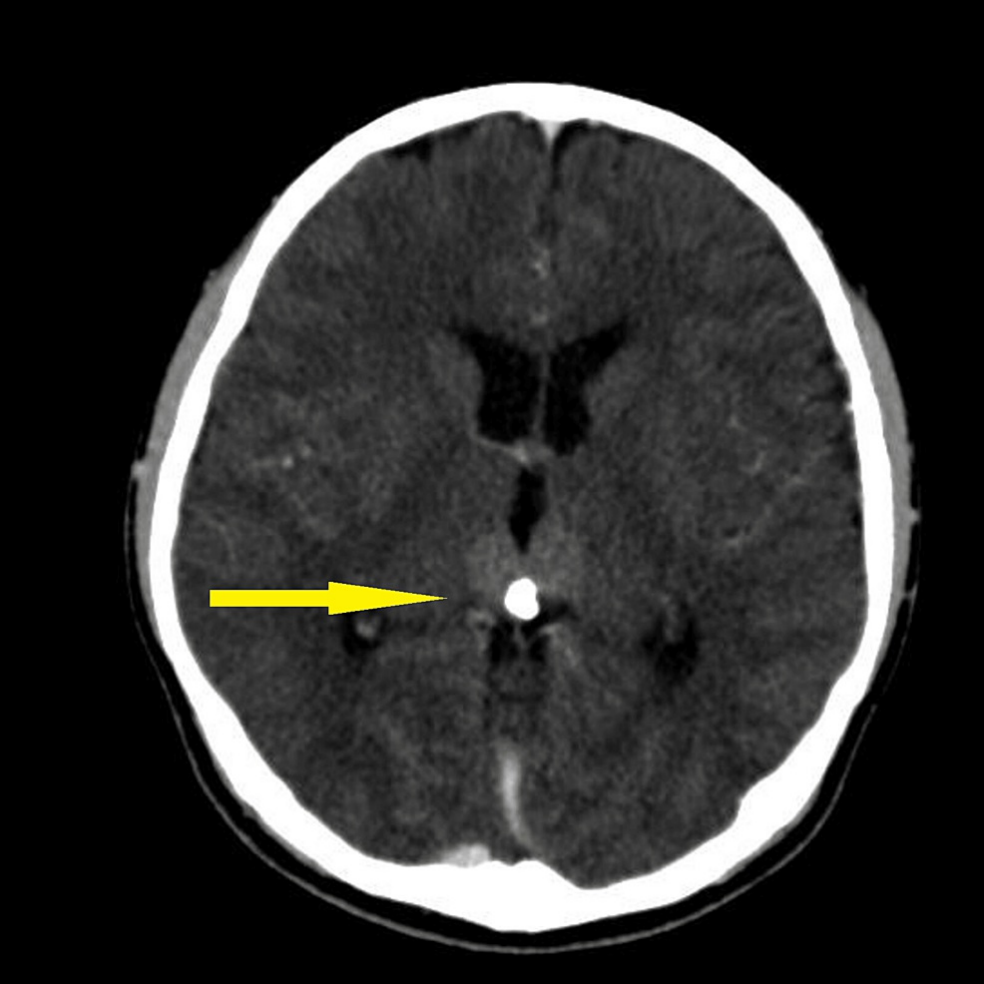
CT brain axial showing hyperdense lesion in the pineal gland region measuring 2.2 cm x 2.6 cm x 1.8 cm (yellow arrow).
